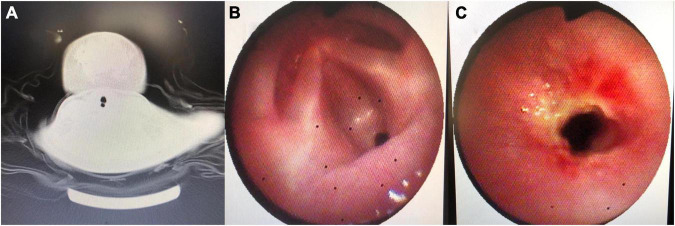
(A) Neck CT noting linear diaphragm in glottis. (B) Image from the first bronchoscopy: webbed connection covering the anterior commissure of glottis and blocking nearly two thirds of glottis. (C) Image from the second bronchoscopy: a small amount of yellow necrotic material attaching to the anterior commissure of glottis.
